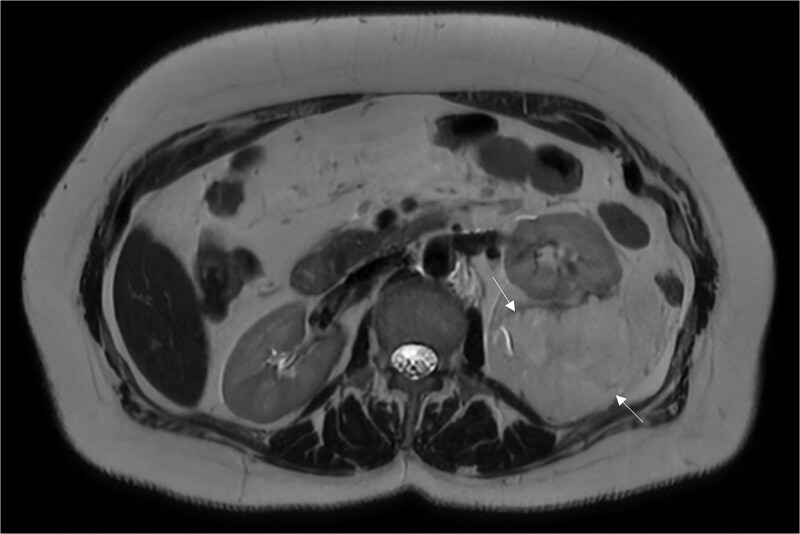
An MRI of the abdomen depicting a large mass in the left posterior pararenal space.
